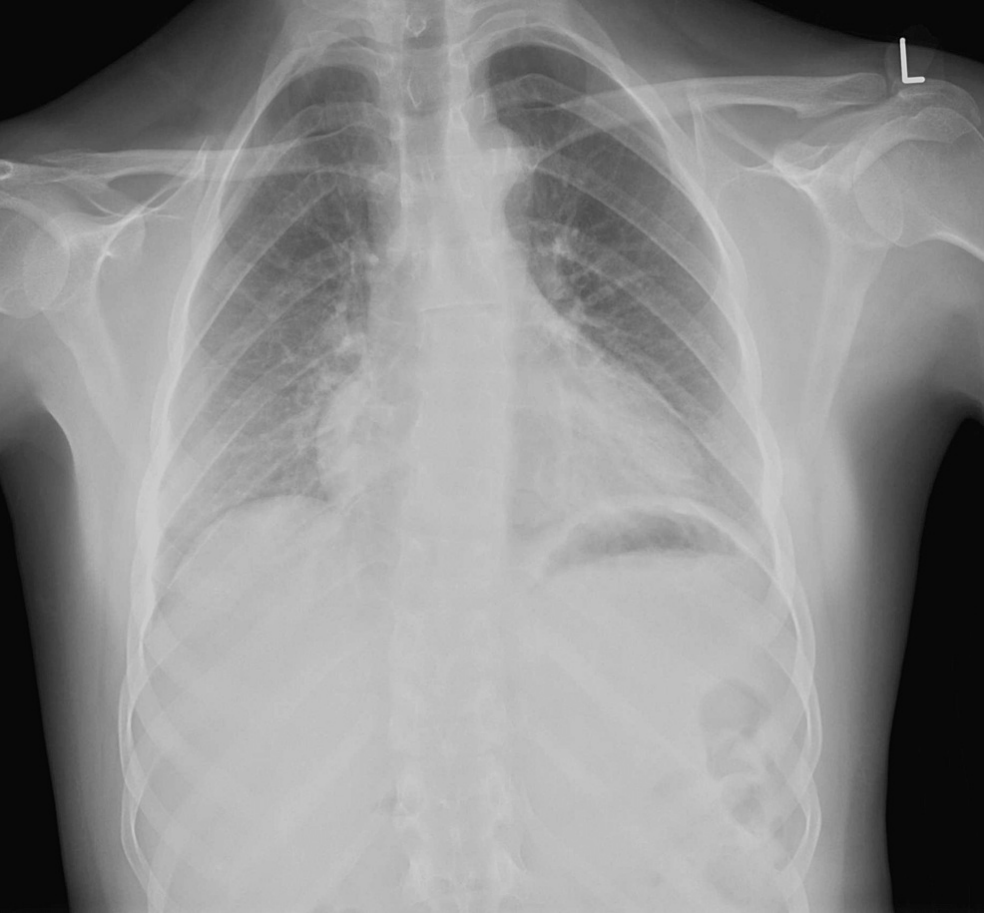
Chest X-ray before the seizure episode.
